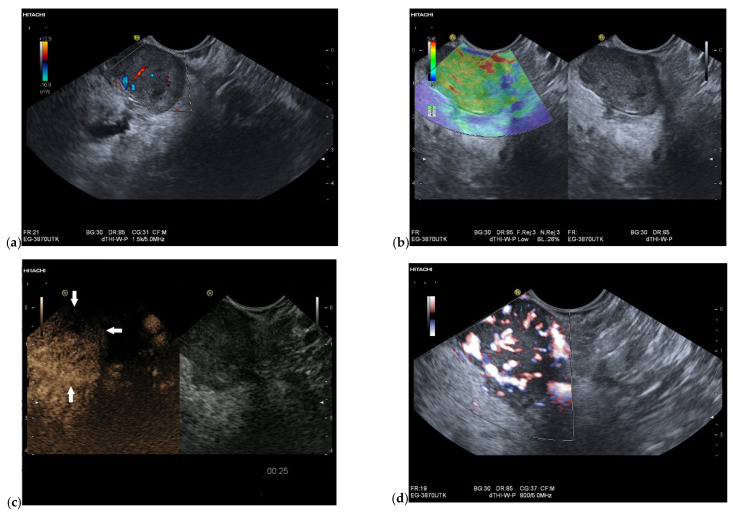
Intrapancreatic metastasis of a RCC in a male, 76 years old. EUS finding of a 25 mm well-circumscribed lesion in the head of the pancreas. The pancreatic duct is not dilated. Vessels are already detectable in the native duplex (a). In the EUS strain elastography, the lesion is softer (b). On CH-EUS with 4.8 mL SonoVue i.v., the lesion is hyperenhanced with a peripheral emphasis and sparing of the central parts (arrows) (c). In contrast-enhanced Power Doppler EUS according to CH-EUS, a large number of vessels are again visible (d).
